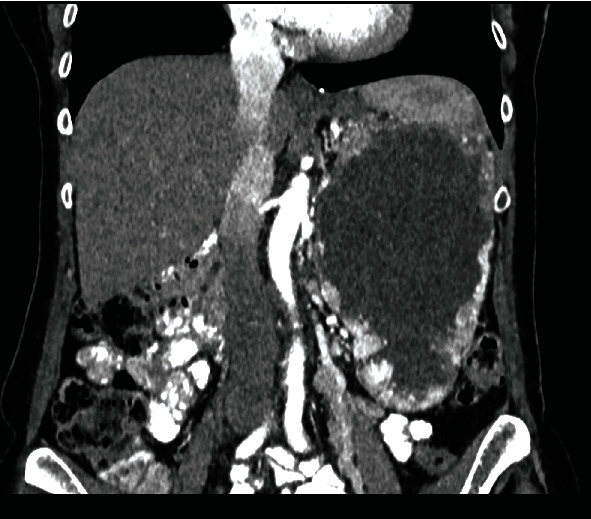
CT showing confirmed a large malignant looking left renal mass with central necrosis.
